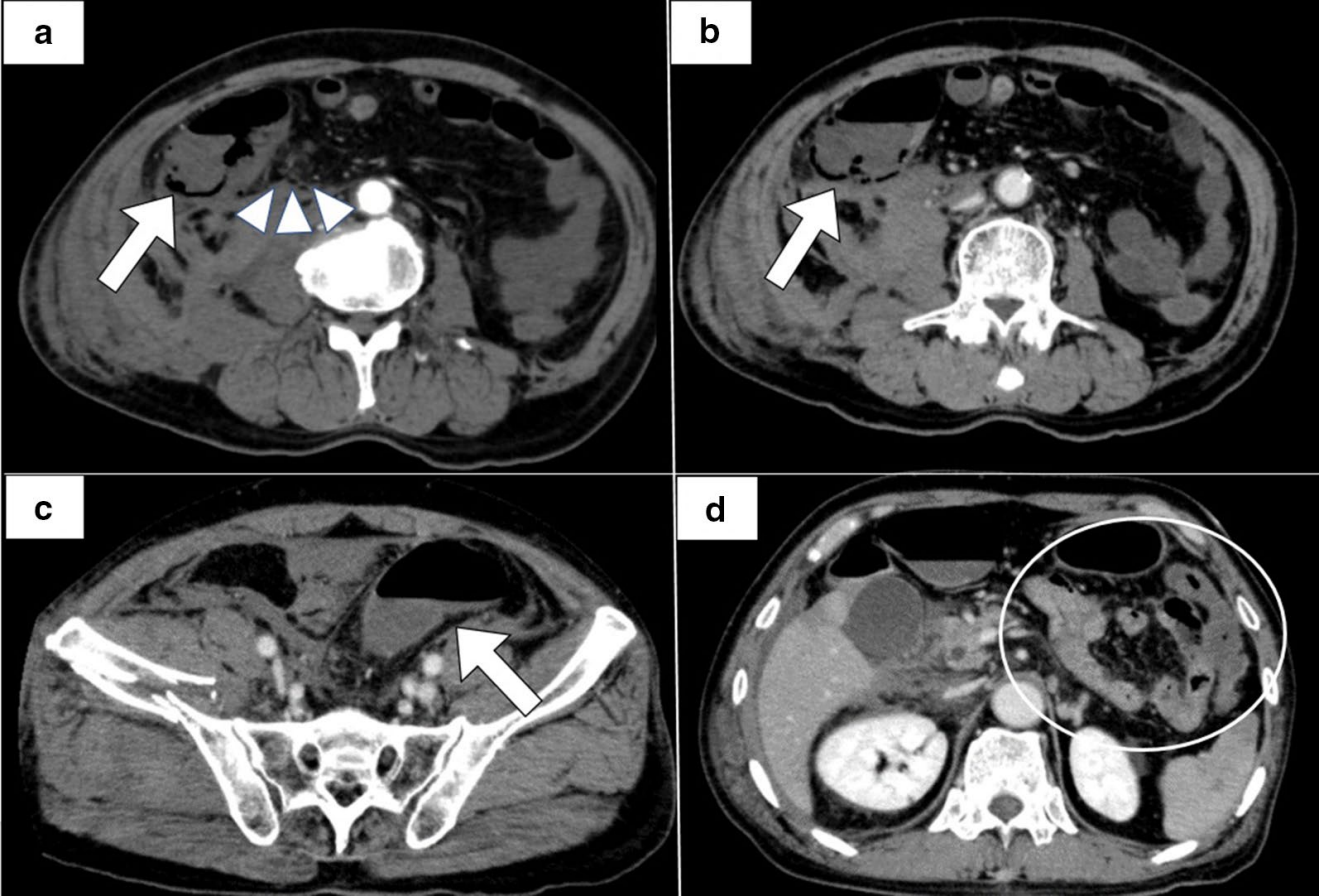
CT findings on day 3: a the early arterial phase; b–d the portal venous phase. a Thickening ascending colon wall, intramural emphysema (arrow), and a slight amount of air in the mesenteric vein (arrowhead). b Intramural emphysema in ascending colon (arrow). c Dilated sigmoid colon (arrow). d Small intestine with no evidence of necrosis (circle)
